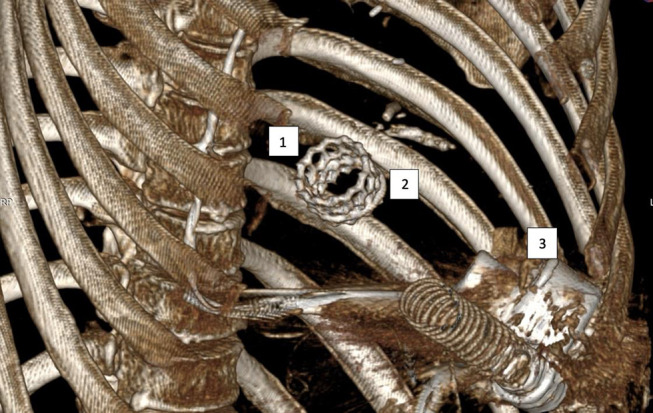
Chest CT after the procedure. 1. AV projection – valve in ring MyVal (Meril) 30.5. 2. The flexible ring Carbomedics annuloflex. 3. LVAD – Heart Mate 3.
